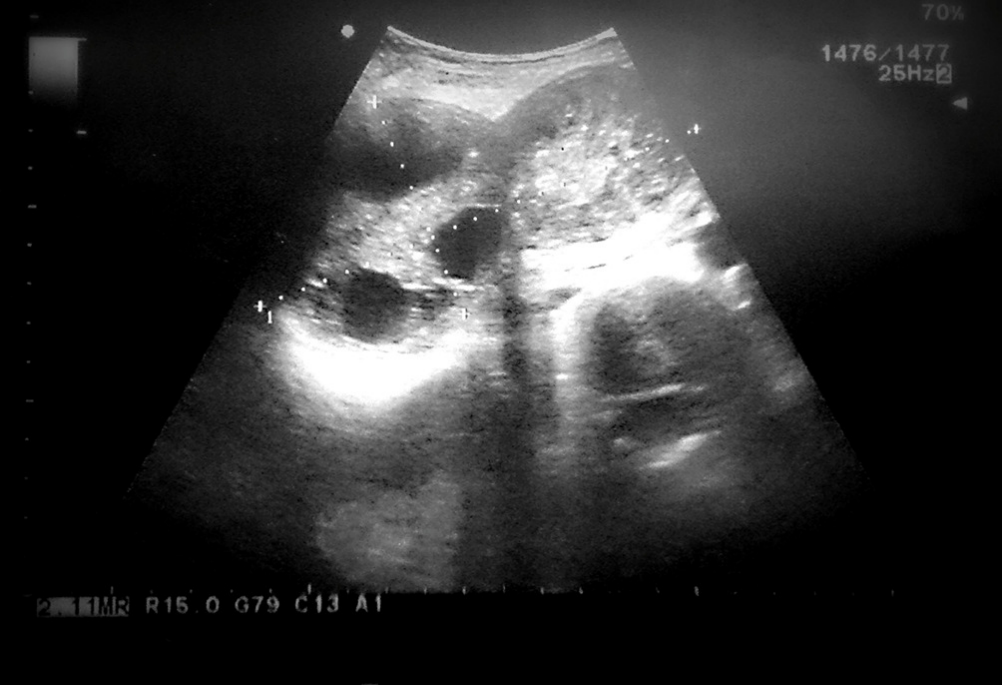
Ultrasonographic imaging showing two adherent heterogeneous lesions in the right hypochondrium, one of which contains multiple daughter cysts arranged at the periphery.
